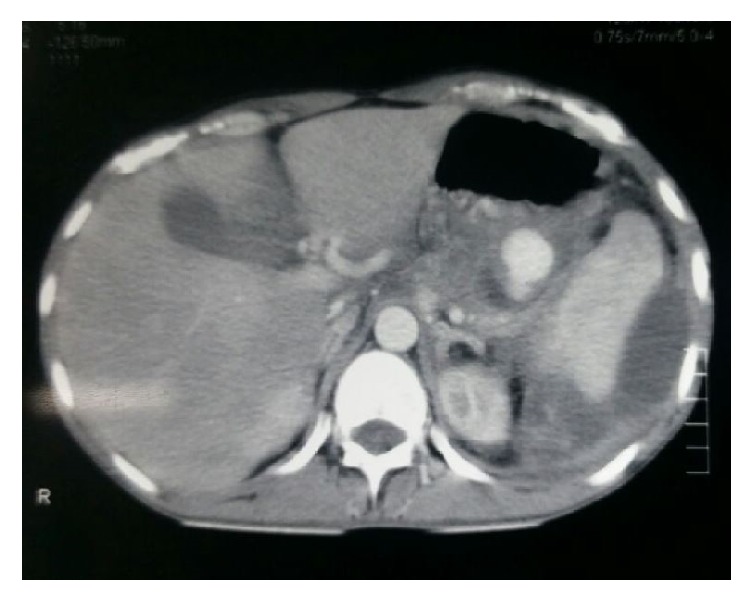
Contrast enhanced CT abdomen showing splenic artery pseudoaneurysm.
